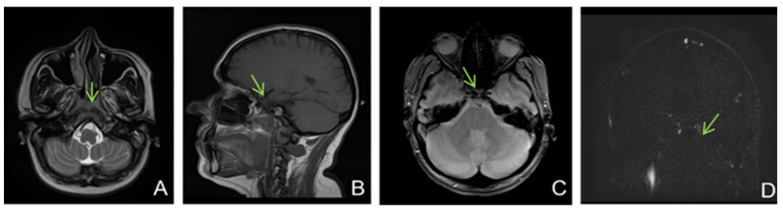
Non-enhanced MRI (at onset): ((A)—T2 axial, (B)—T1 sagittal, (C)—T2* axial, (D)—venous TOF coronal) shows unremarkable parenchyma and absence of flow in cavernous sinuses on TOF imagesFive months later, the patient came back to our clinic complaining of paroxysmal episodes of altered state of consciousness with language disorders such as verbal barrage followed by disorientation. An EEG was performed, showing a low-voltage background path, weakly modulated in spindles in the left derivations, reactive when opening the eyes, and rare isolated degraded peak-wave complexes, thus concluding that the patient was experiencing focal onset impaired awareness seizures; she was then started on antiepileptic treatment with oxcarbazepine 300 mg twice daily. She also was psychiatrically examined due to emotional lability confirming the diagnosis of depression and allowing antidepressant treatment to be started.
